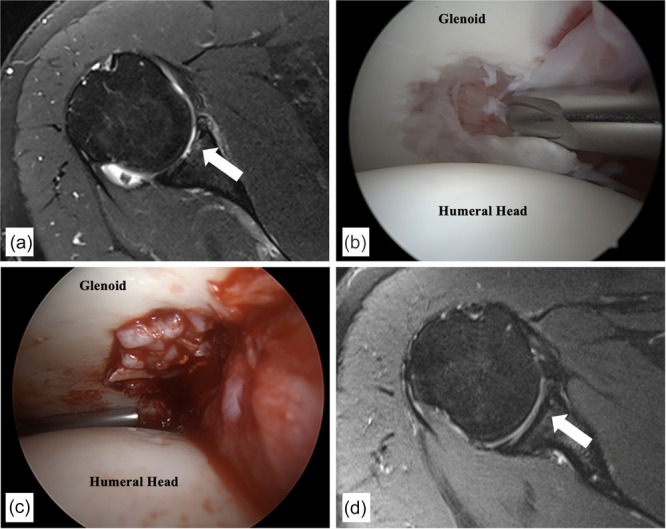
Preoperative MRI scan in the axial plane of Patient ID 3 showing a cartilage defect at the anterior glenoid surface (a). The MRI, 31 months after MCI, shows successful defect coverage (d). Intraoperative images of the same patient demonstrating the cartilage defect prior to debridement (b) and after MCI (c). MCI, minced cartilage implantation; MRI, magnetic resonance imaging.
